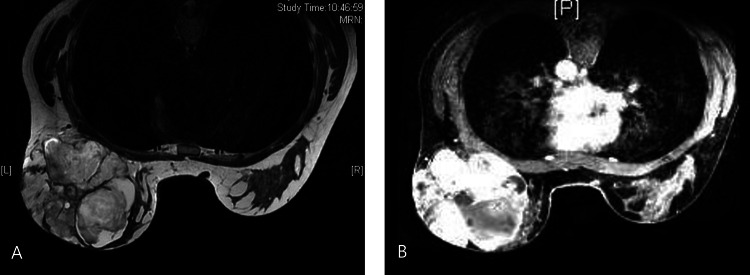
(A, B) Breast MRI showing a large tumor with irregular walls with T1-weighted image with high intensity and T2 image with low intensity. A rapid enhancement pattern is evident.
